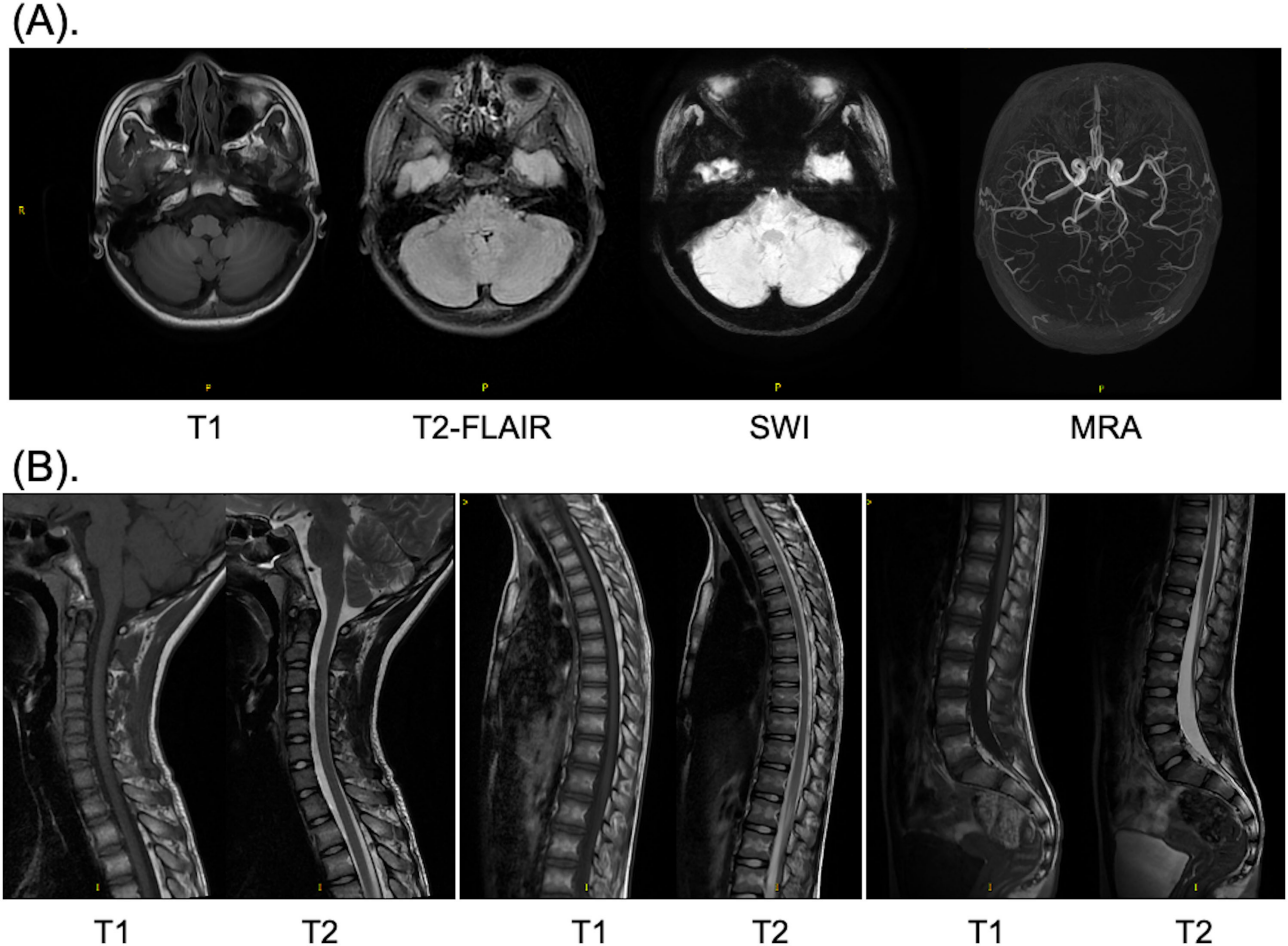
MRI images of the brain (A) and whole spine (B) after admission. (A) T1 weighted, T2 weighted, SWI and MRA scan of the brain; (B) T1 and T2 weighted scan of the cervical, thoracic, and lumbar vertebrae. MRI, magnetic resonance imaging; SWI, susceptibility weighted imaging; MRA, magnetic resonance angiography.
